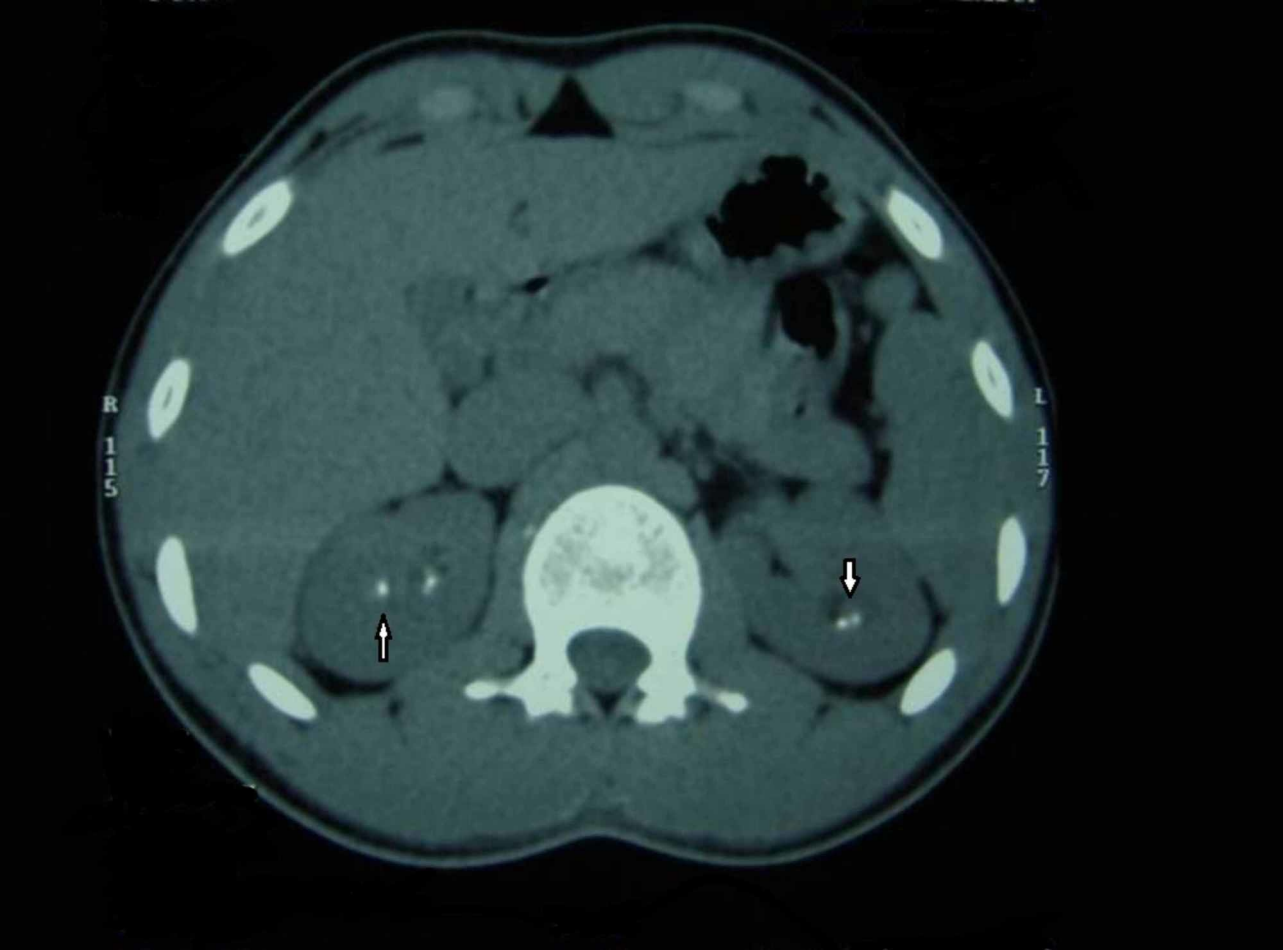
Axial CT scan of kidneys showing bilateral nephrocalcinosis (male patient)
